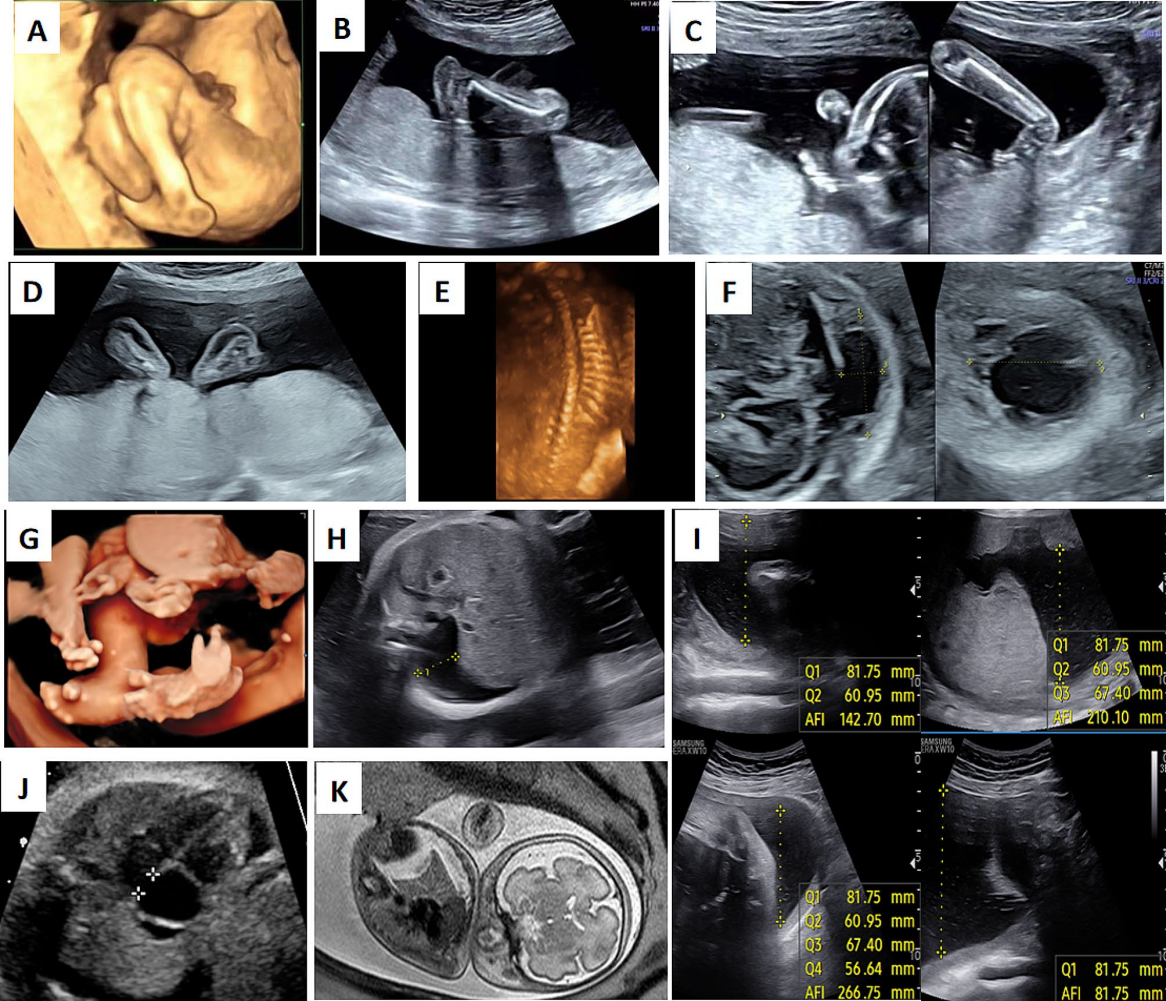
Imaging findings. (A-C) fetal ultrasound images from F1-II0.3. (A) ankle and knee contractures; (B) the left foot presented with rocker bottom deformity; (C) wrist contractures. (D-F) Fetal ultrasound images from F2-II0.2. (D) the abnormal fetal posture with the palms of the feet facing each other; (E) the abnormal curvature of the fetal spine; (F) thickened nuchal fold with cystic the dark area inside. (G-K) Imaging findings from F3-II0.1. (G) flexed position of all limb joints; (H) a right pleural effusion with a width of about 16 mm; (I) mild pericardial effusion with a width of 3.6 mm; (J) widened extracerebral space and mild deformation of the cerebral parenchyma showed by MRI; (K) polyhydramnios with an amniotic fluid index (AFI) of 266 mm and a maximum depth of 95 mm
